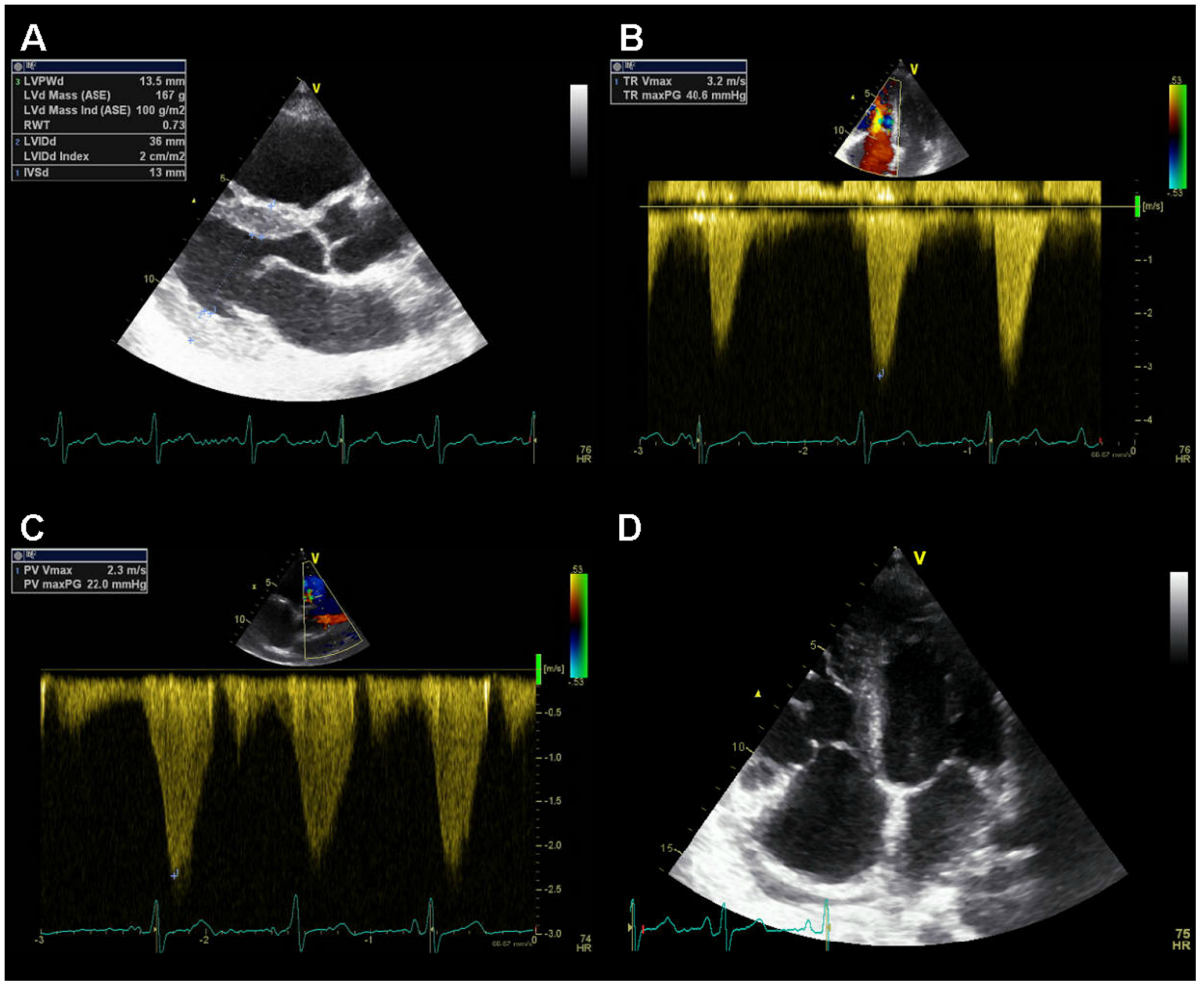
Example of echocardiographic characteristics of a patient with carcinoid heart disease. A: Parasternal long axis view showing thickened LV walls (inter-ventricular septum 13 mm, posterior wall 13 mm). B: Continuous wave Doppler of the tricuspid valve jet. The peak velocity (41 mm Hg) indicates severe tricuspid insufficiency. C: Continuous wave Doppler of the pulmonary valve. The peak gradient (22 mmHg) indicates mild pulmonary valve stenosis. D: 2D four chamber image. On the right in the image normal function of the mitral valve. On the left in the image retraction and immobilisation of the tricuspid valve. The leaflets of the tricuspid valves are also hyperechogenic than normal.
